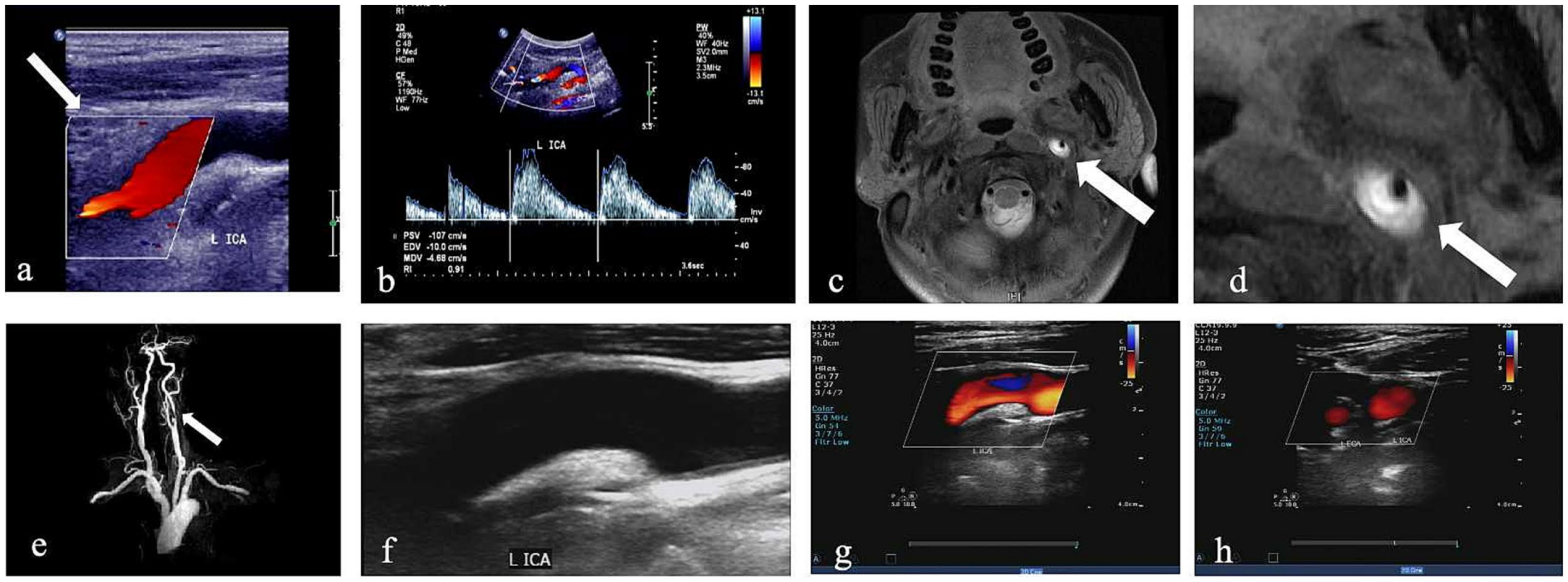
CDU imaging for one patient with left internal CAD. (a,b) Greyscale and pulsed-wave Doppler with hypoechogenic intramural hematoma (white arrow) and lumen narrowing. (c,d) Corresponding HRMRI with evidence of a crescent-shape hyperintense on axial FS-T2W images and enlarged view of FS-T2W (white arrow). (e) 3D time-of-flight (TOF) magnetic resonance angiography imaging. (f–h) CDU done 6 months later shows recanalization after treatment; the wall structure of the ICA returned to normal, and the blood flow signal was completely filled.
